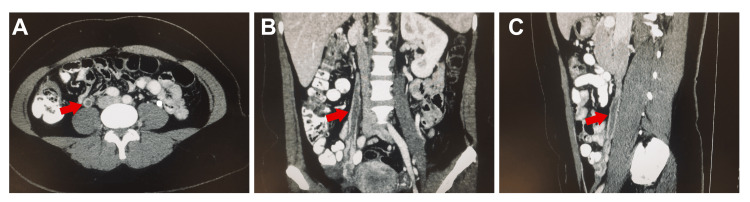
A) Axial, B) Coronal, C) Sagittal enhanced-CT views of right ovarian thrombosis (red arrows) A low-attenuation filling defect is present within the dilated vein.
